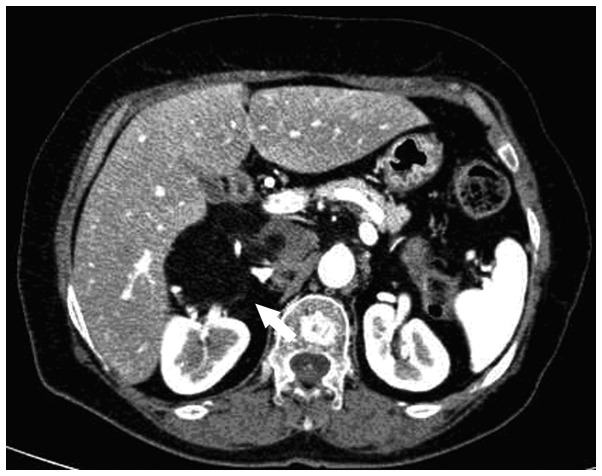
Contrast multi-slice computed tomography scan revealed a right fat-containing renal mass with renal vein and vena caval extension.
